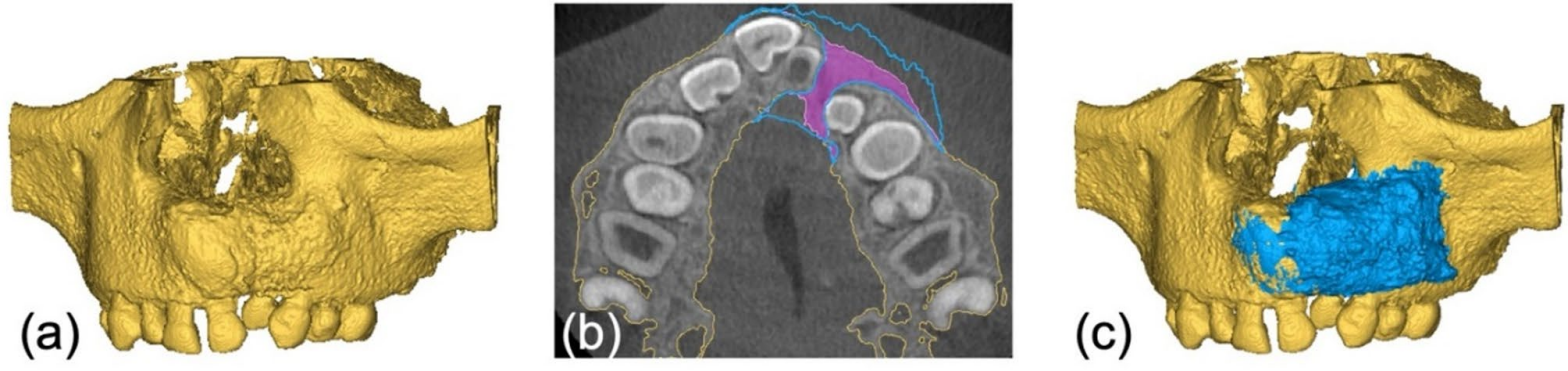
Model V2 from 6 months postoperative CBCT (a), V_graft_ (blue) superimposed on V2 (yellow), and V_integrated bone_ (pink) which is the overlap between V2 (yellow) and V_graft_ in axial view (b), V_graft_ superimposed on V2 (yellow) in 3D view (c).
